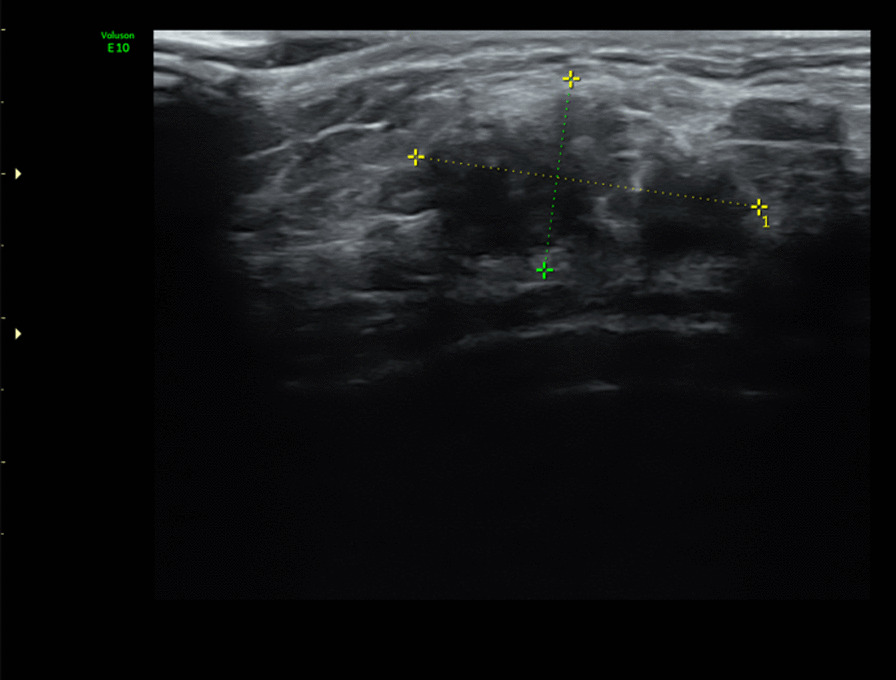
Ultrasound image of the right breast mass showing a heterogeneous hypoechoic nodule, approximately 24 × 13 mm in size, with heterogeneous internal echo, posterior echo attenuation, and poor blood supply, with an ultrasound rating of BI-RADS 4B
